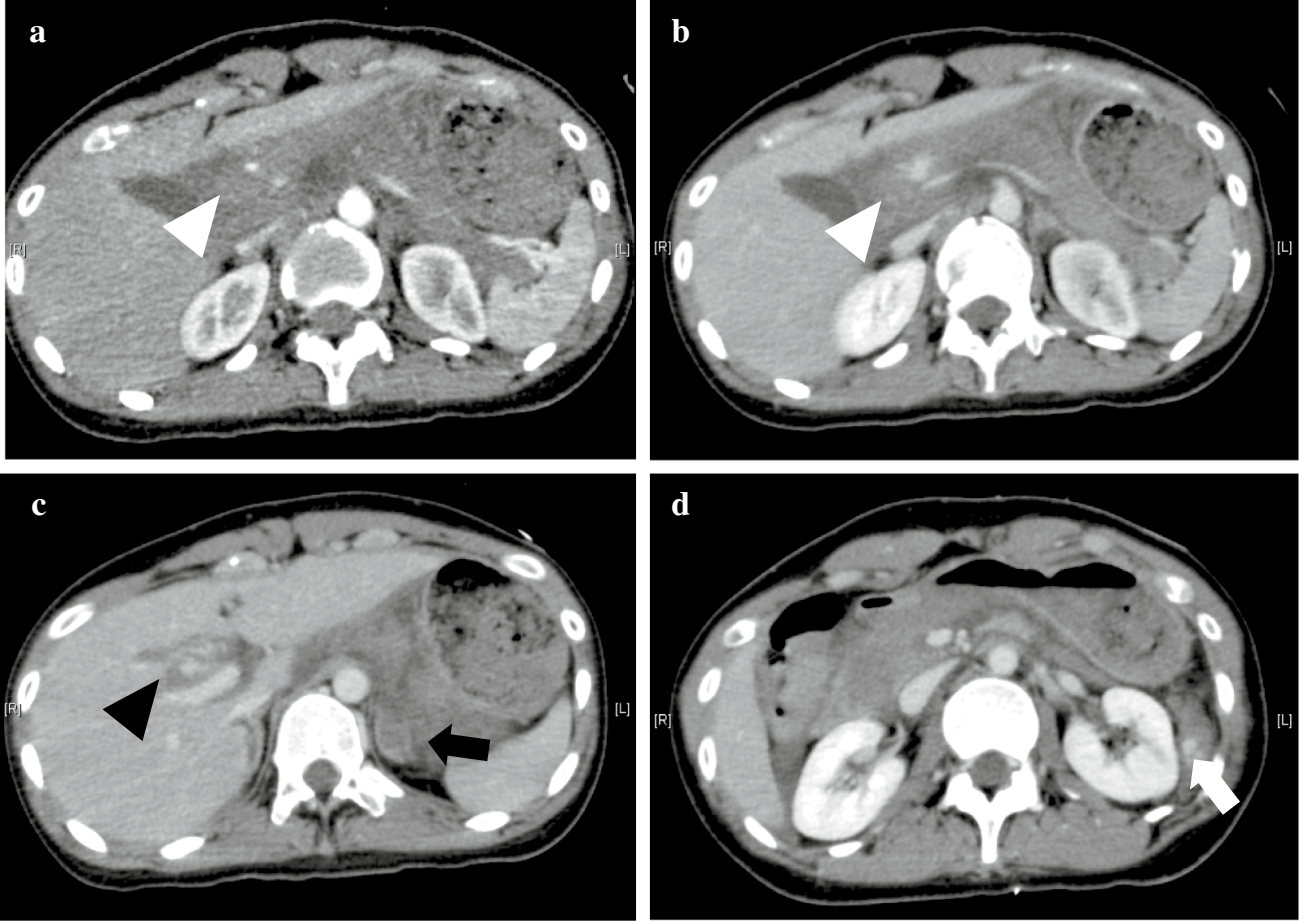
Computed tomography findings on admission. a, b Abdominal computed tomography scan revealing pancreatic head and body injury with hematoma and extravasation of contrast media (white arrowhead). c, d Abdominal CT showing liver injury (black arrowhead) and lower lobe of splenic injury (white arrow) with extravasation of contrast media and left adrenal injury (black arrow)
